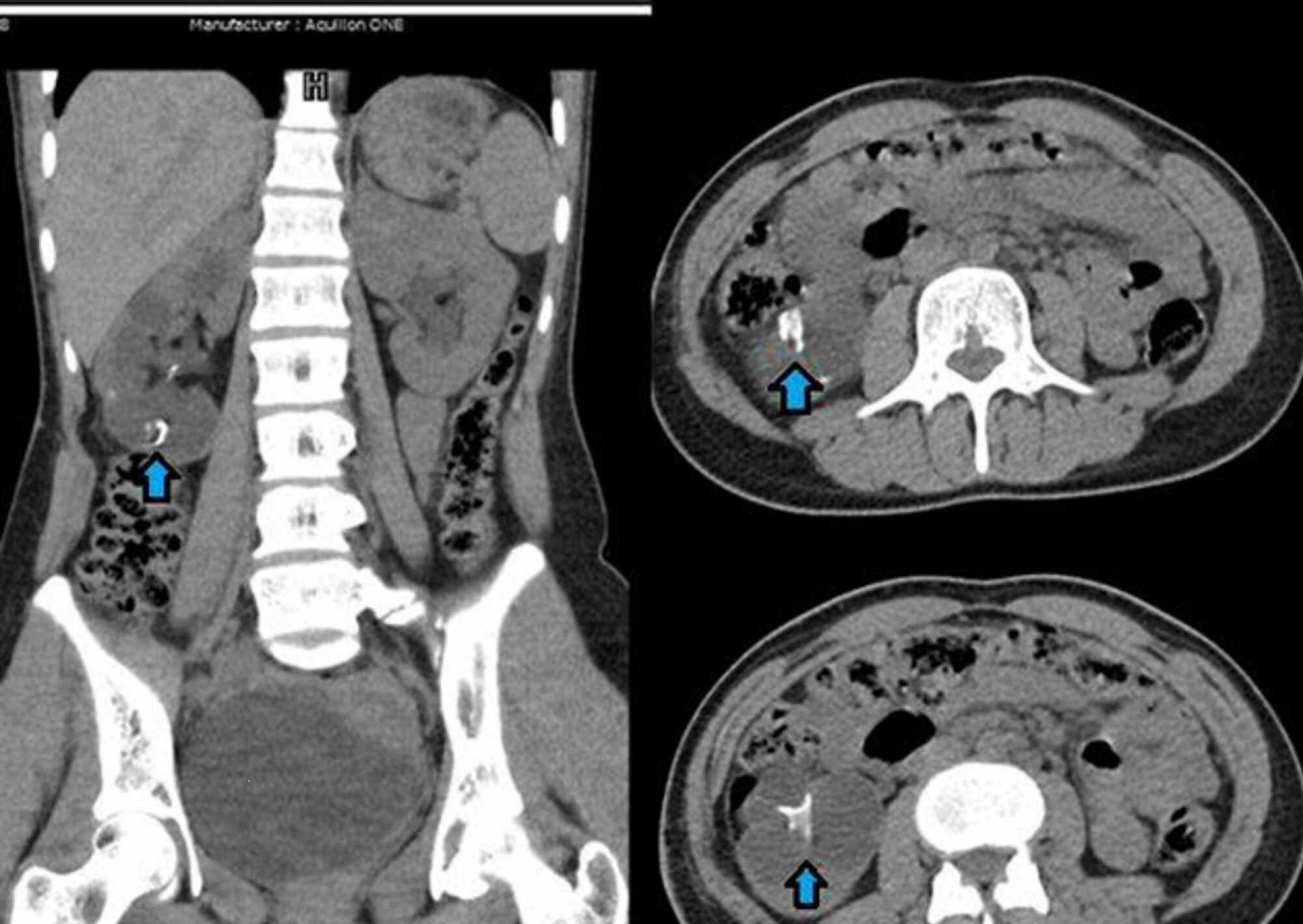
Coronal and Axial images of CT KUB (kidney, ureter, and bladder) X-ray: showing hyperdense circular density in lower pole of right kidney (blue arrow)
